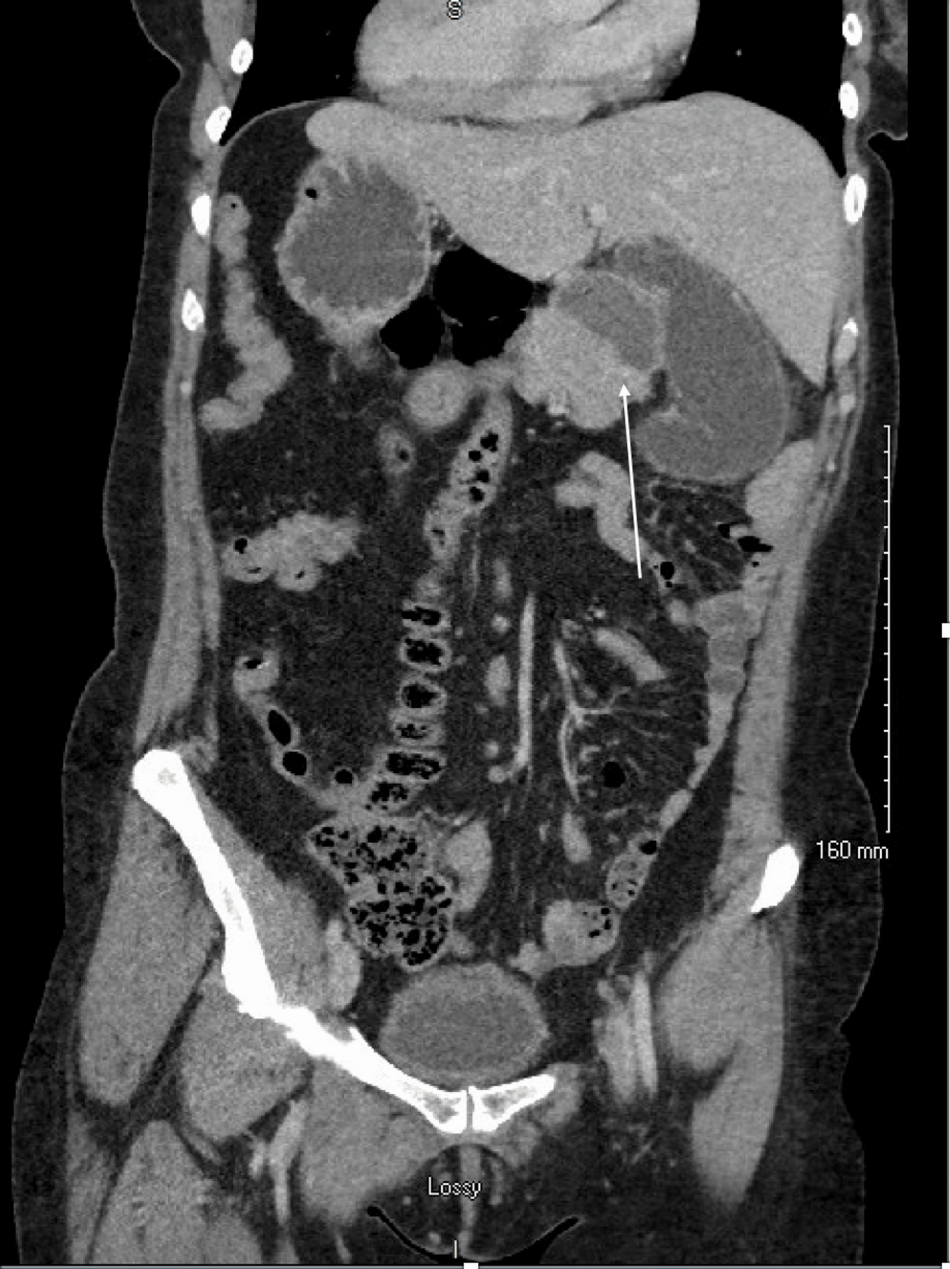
Coronal view of CT abdomen and pelvis with contrast consistent with SIT. The white arrow indicates duodenal intussusception. Note: The liver is located in the left upper quadrant SIT: situs inversus totalis
